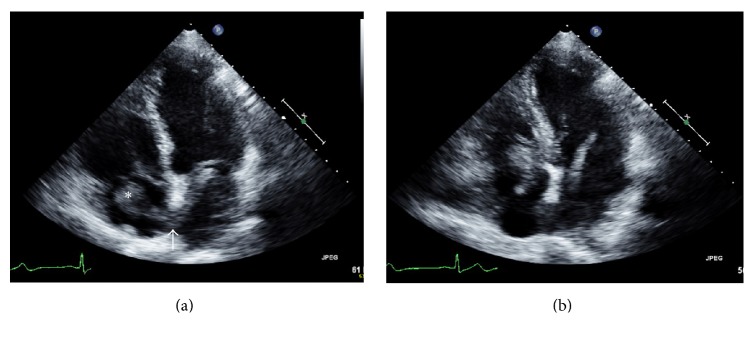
(a) Transthoracic echocardiogram apical four-chamber view in diastole showing a large thrombus (asterisk) extending from the right atrium to the left atrium via a patent foramen ovale (arrow). (b) Apical four-chamber view in diastole demonstrating prolapse of the thrombus across the mitral and tricuspid valves.
